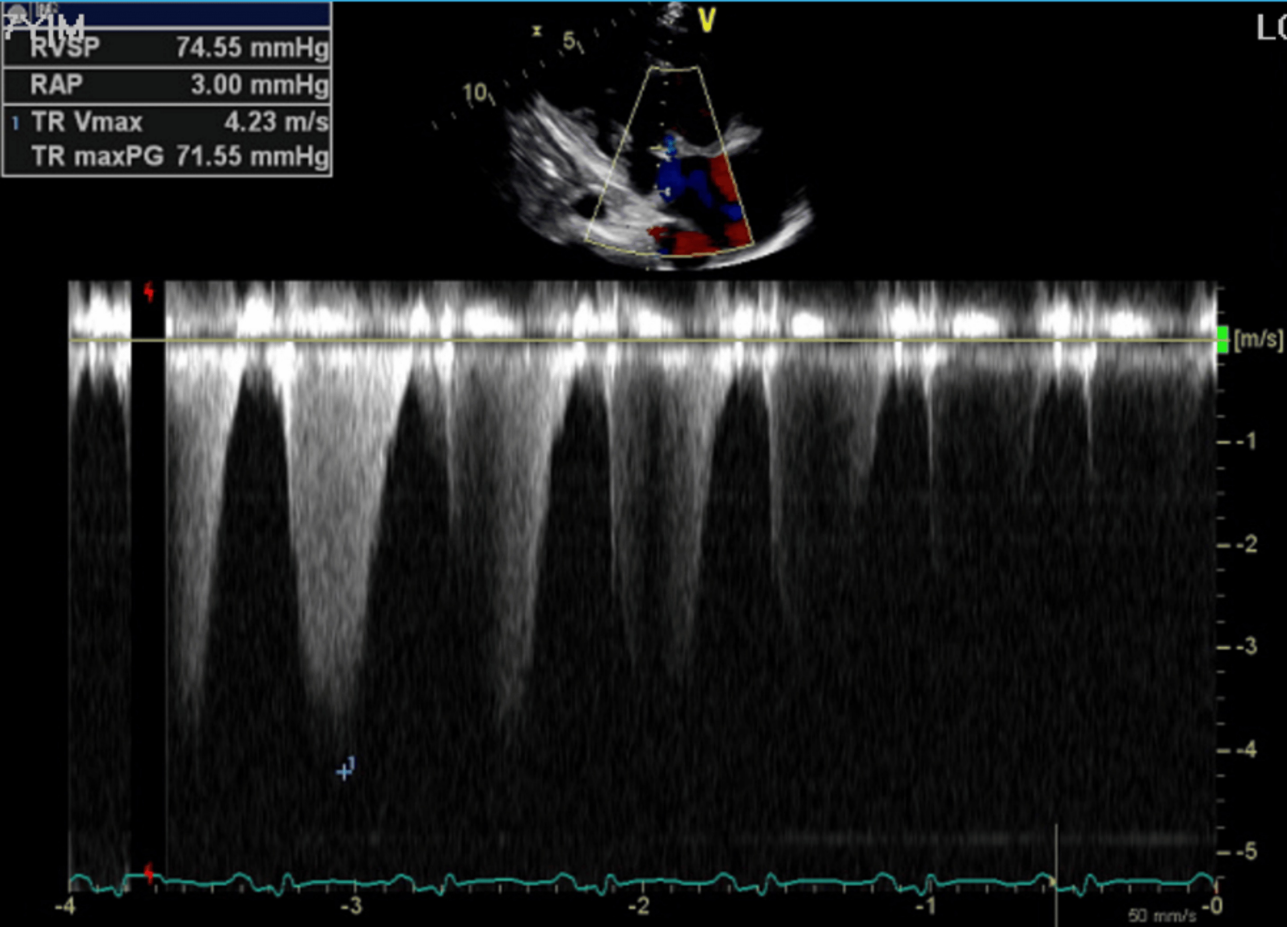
Echocardiogram showing elevated right ventricular systolic pressure (RVSP), indicative of pulmonary hypertension.
